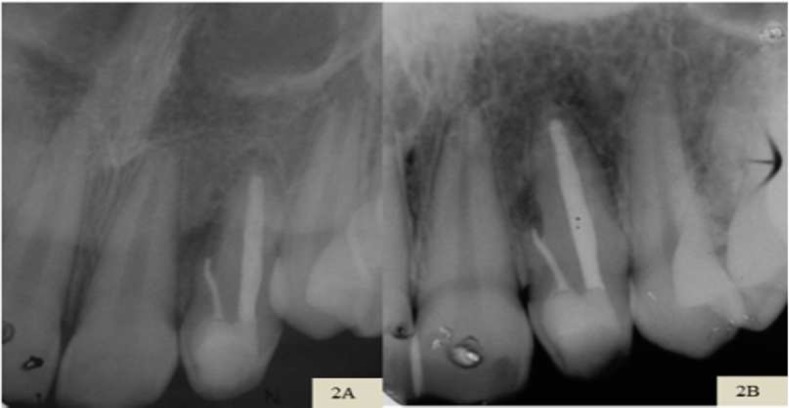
A)* Periapical follow-up radiography after 1 year of endodontic treatment; demonstrating a decrease in periapical lesion; *B)* After 3 years; exhibiting bone neoformation
